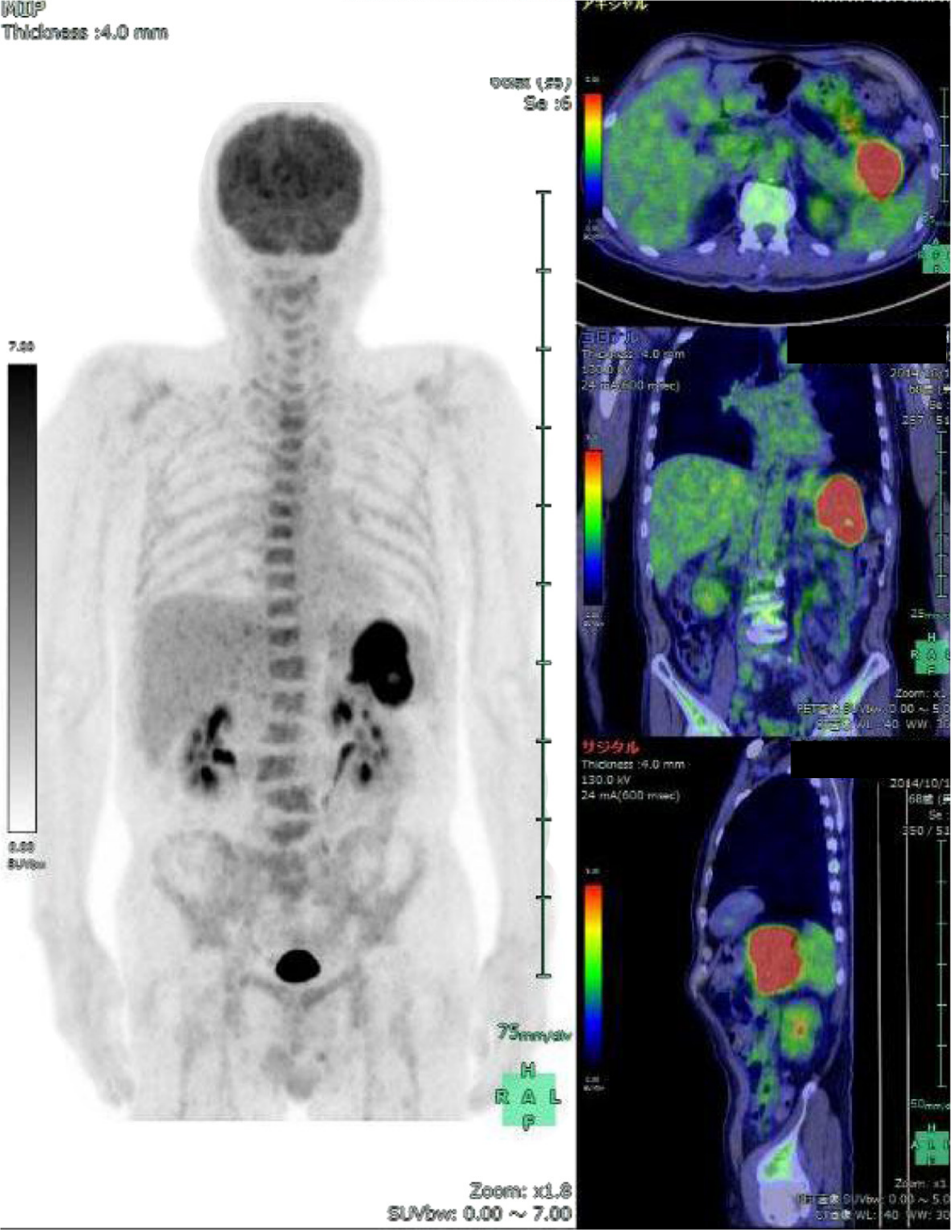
FDG PET ^18^ F-2-fluoro-2-deoxyglucose positron-emission tomography/computed tomography (FDG PET/CT) showed FDG accumulation in the tail of the pancreas (SUV, 17.1)
